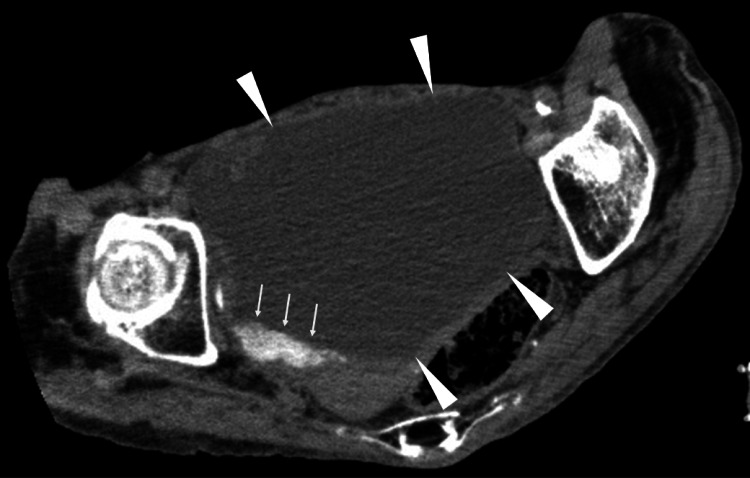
Markedly distended bladder with urinary stasis Abdominal computed tomography demonstrates a markedly distended bladder with significant urinary stasis (arrowheads) and abundant intravesical sediment (arrows). These findings are consistent with chronic voiding dysfunction and obstructive urinary retention.
